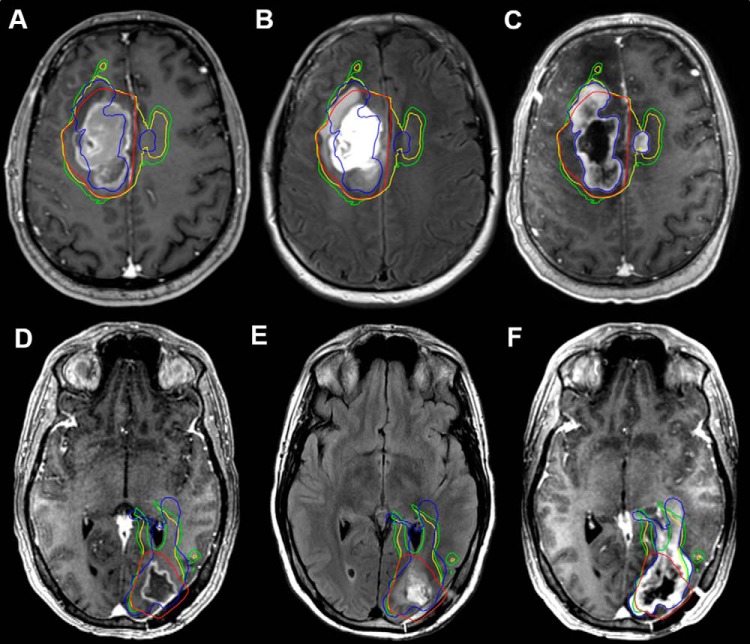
PreRT CE-T1W, PreRT T2-weighted/FLAIR, and recurrence CE-T1W images with conventional, 1.75-fold, and 2.0-fold CTVs (red, green, and yellow contours, respectively) for patients 4 (A–C) and 11 (D–F). Regions of tumor recurrence (blue contour) anterior and contralateral to contrast enhancement in patient 4 (A) and passing toward the ventricle in patient 11 (D) were not encompassed by the CTV60. The fusion of 1.75- and 2.0-fold Cho/NAA abnormalities with the CTV60 resulted in a target covering a significantly larger proportion of the recurrence.
